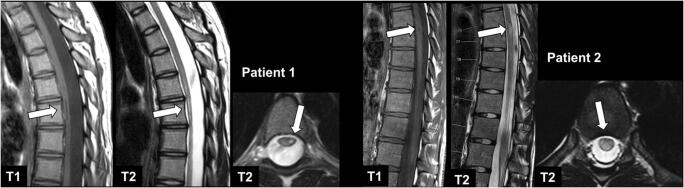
MRI of a 19-year-old female patient with NMOSD (patient 1) and a 20-year-old female patient with relapsing-remitting MS (patient 2) with sagittal T1-w, sagittal T2-w, and axial T2-w images (from left to right). Note the long-segment T1-w hypointense lesion in the thoracic spinal cord on the left side of patient 1 with concomitant atrophy of the spinal cord and the focal T1-w hypointense lesion in patient 2 (arrows), compatible with black holes
